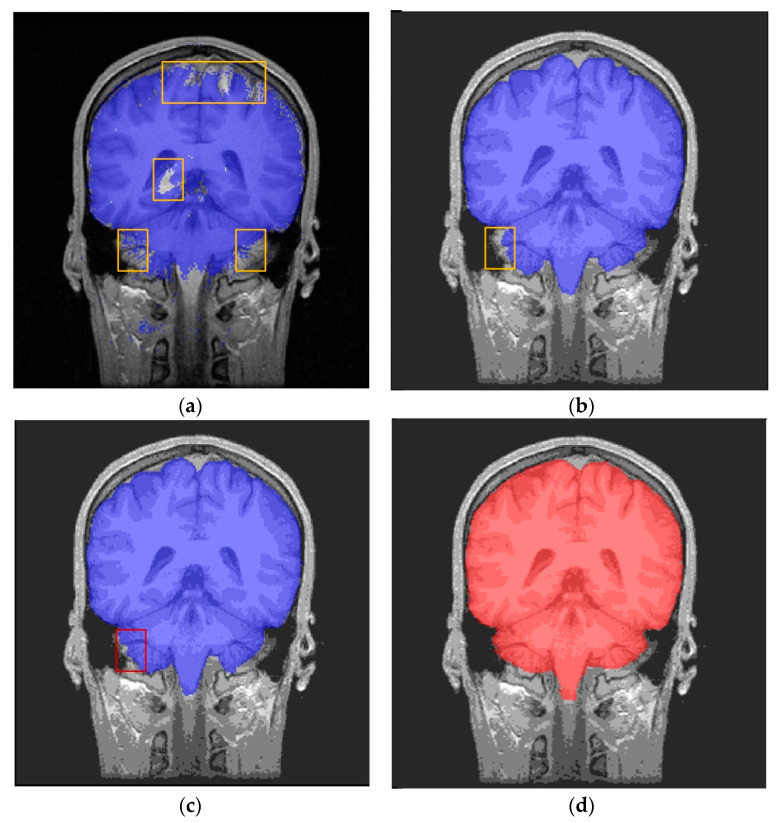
The predicted masks superimposed on MRI data in the coronal plane. These four images show the improvement of the predicted brain mask in different steps of the proposed algorithm. (a) The predicted brain mask with the original U-Net, and several deficiencies are marked with boxes in yellow. (b) The prediction performance is improved with K-means preprocessing, whereas one can still see the drop-off at the edges of the brain. (c) The image demonstrates the enhancement of the edge area through the utilization of a hybrid net, which can be seen within the red box. (d) The overlay of the referenced label mask onto the MRI data.
